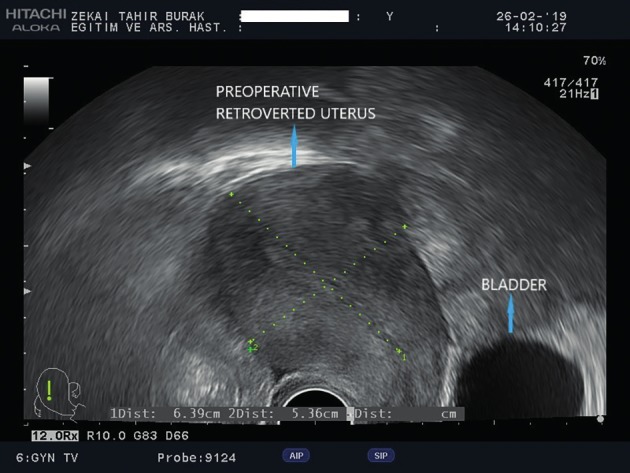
Preoperative transvaginal ultrasonographic view of retroverted uterus.
